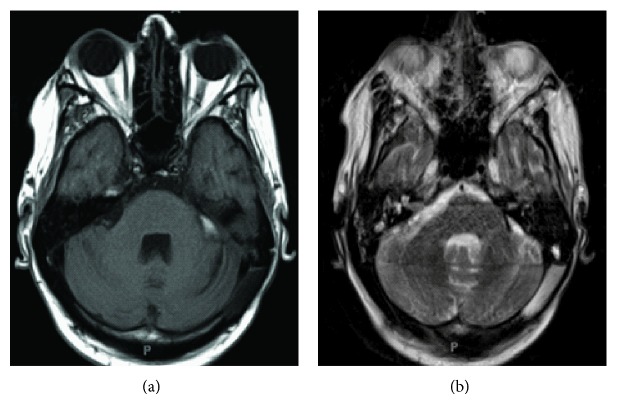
(a) T1-axial MRI with contrast and (b) T2-axial Flair MRI demonstrating interval decrease in size of previously identified enhancing mass in the left cerebellopontine angle after resection.
